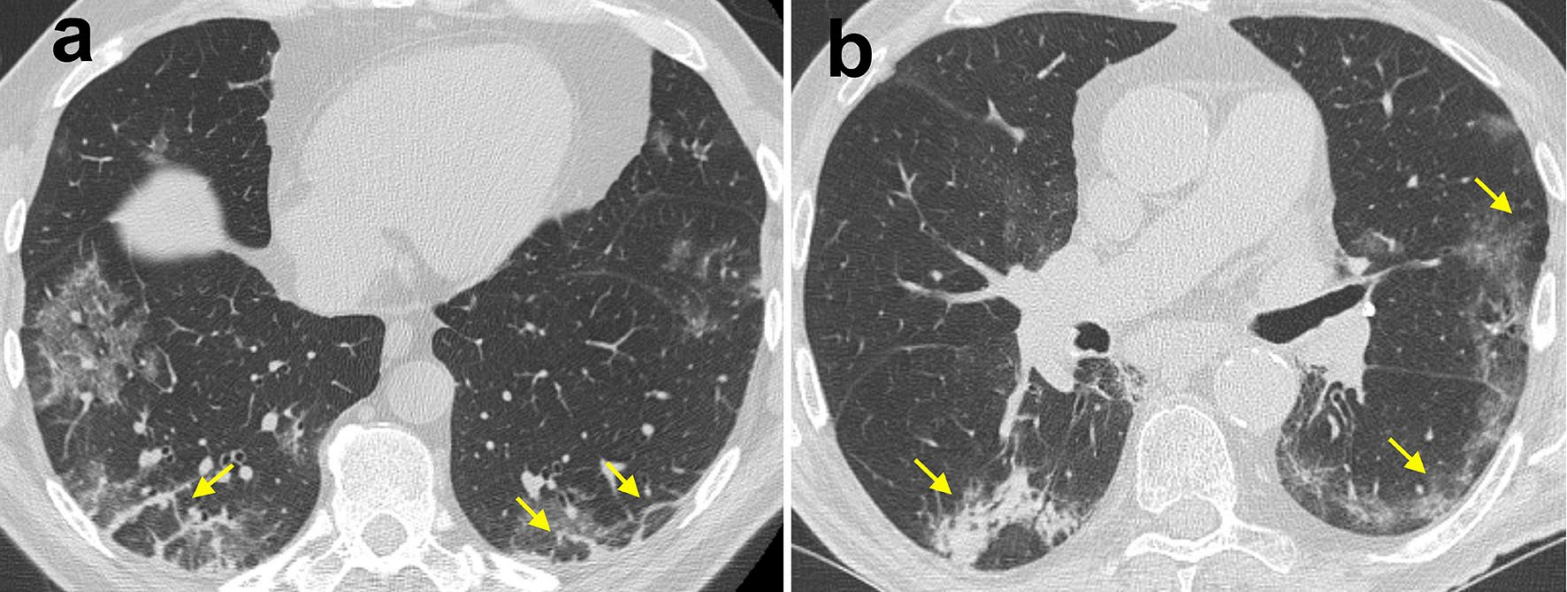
Organizing pneumonia pattern of lung injury in COVID-19 pneumonia. High-resolution CT images of a male COVID-19 patient presenting fever with cough and dyspnea for 15 days (a) and of a different male COVID-19 patient 14 days after the clinical onset (b) show perilobular thickening and parenchymal opacities (both ground-glass opacity and consolidation), along with superimposed signs of distortion, in the posterior subpleural regions of both lower lobes (arrows)
